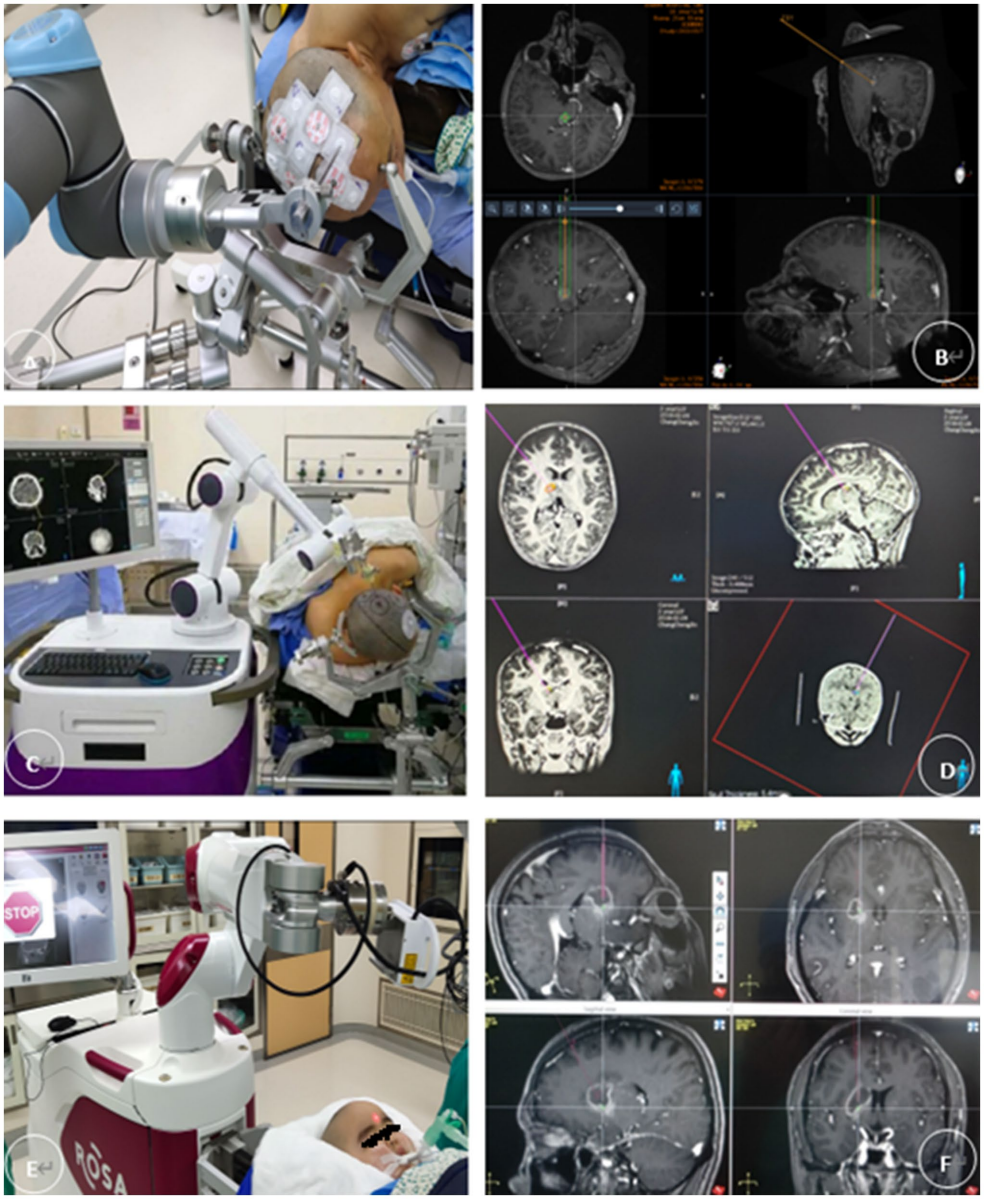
(A,B) The REMEBOT and (C,D) the CAS-R-2 use scalp markers for registration. The patient’s head is prepared for skin adhesion to the scalp markers before surgery (E,F). The ROSA robot uses facial laser scanning for registration; it automatically performs a face scan followed by image reformation. Magnetic resonance imaging and computed tomography of the head are performed and fused with the surgical planning procedure, and the images are transmitted to the surgical robotic procedure planning system. This system is capable of establishing and maintaining a case history, entering and demonstrating data in Digital Imaging and Communications in Medicine format, performing three-dimensional reconstruction for visualization, and planning the puncture tract. The patient’s head is fixed with a plastic pillow or Mayfield head frame after induction of general anesthesia. The mechanical arm substitutes for a stereotactic arc and is used to hold the probe with which the surgeon finally performs the stereotactic procedure. The surgeon performs depth measurements using the depth of the probe.
